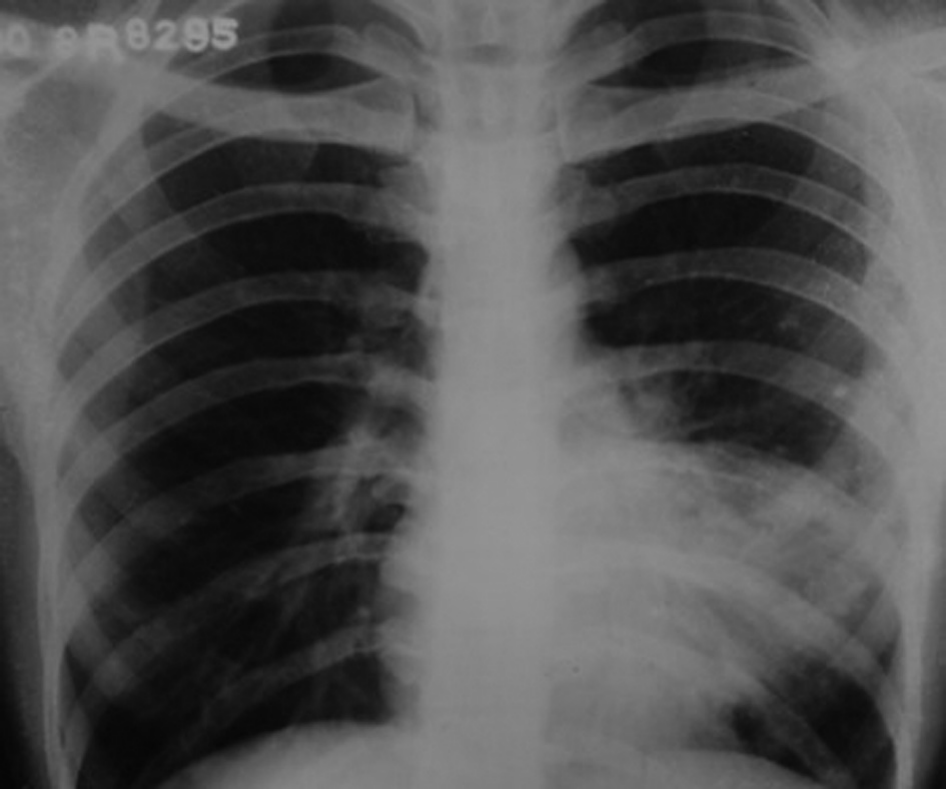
Figure 1. Chest radiograph three years prior to admission shows a large ill-defined opacity with multiple lucencies adjacent to the left heart border
